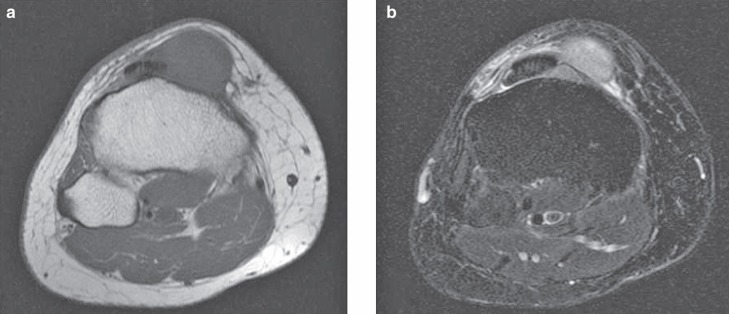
Magnetic resonance images of the tumor at the initial presentation. The tumor showed low signal intensity on T1-weighted images (a) and high signal intensity on fat-suppressed T2-weighted images (b). The tumor was located closely to the patellar tendon, and a part of the tumor crept beneath the tendon. A high signal intensity area, suggesting edema, was seen around the tumor.
